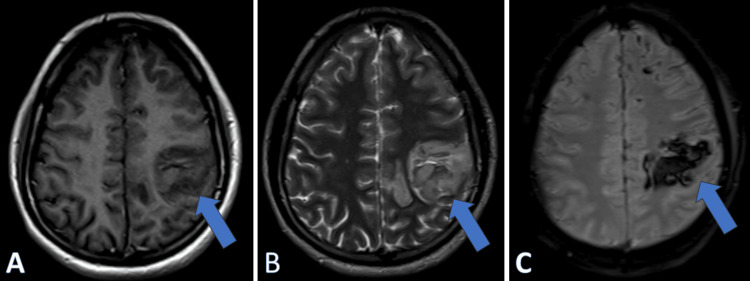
MRI brain in axial view reveals a well-defined area (blue arrows) of size 34 x 40 x 33 mm (CC X AP X TR) in the left frontoparietal region, showing heterogenous hyperintensity in T1 WI (A) and T2 WI (B) and blooming on GRE sequence (C) MRI: magnetic resonance imaging, CC: craniocaudal, AP: anteroposterior, TR: transverse, WI: weighted imaging, GRE: gradient echo recall
